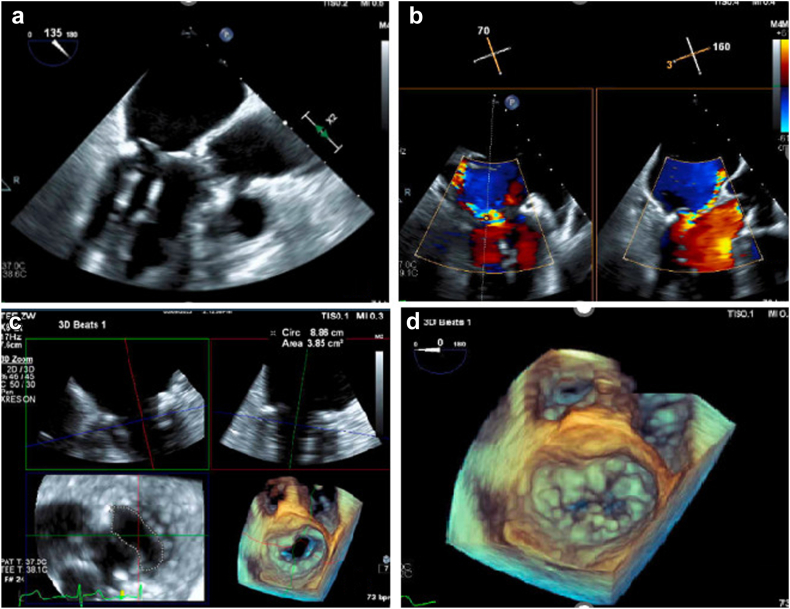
(a) TEE LAX view showing flail P2 segment with torn chordae and MAC extending to leaflets and subvalvular apparatus. (b) Color Doppler in LAX and commissural views showing severe eccentric MR. (c) 3D mitral valve reconstruction showing concentric MAC and valve area of 3.8 cm^2^. (d) P2 prolapse on 3D reconstruction (surgeon's view). Abbreviations: MAC, mitral annular calcification; MR, mitral regurgitation; TEE, transesophageal echocardiography.
